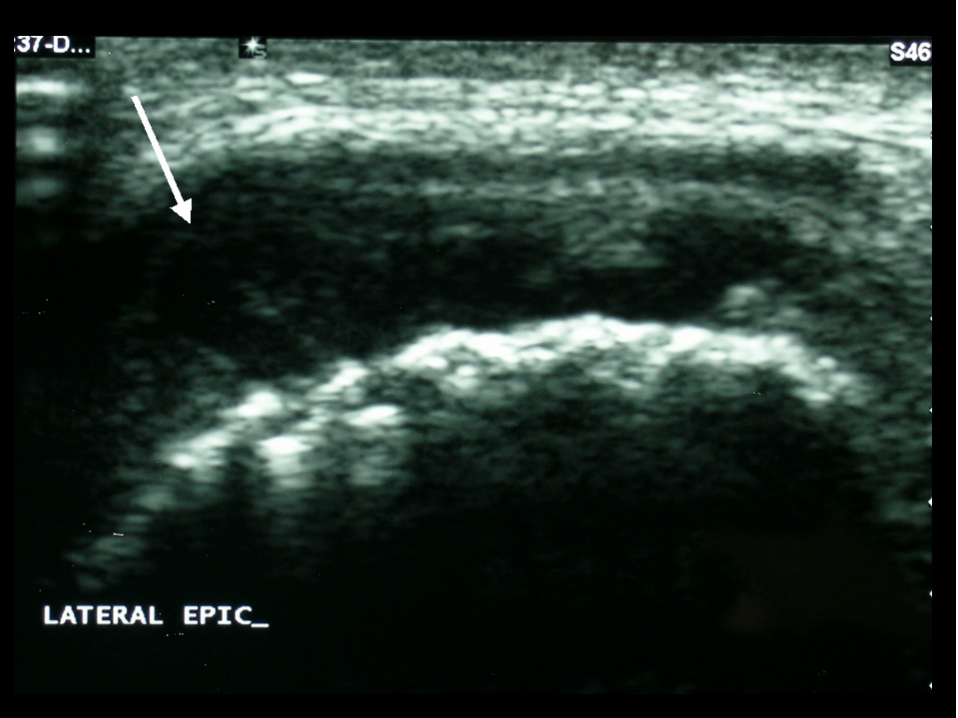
Ultrasound examination of the right elbow. This confirms the presence of a hypoechoic joint effusion (arrowed).
